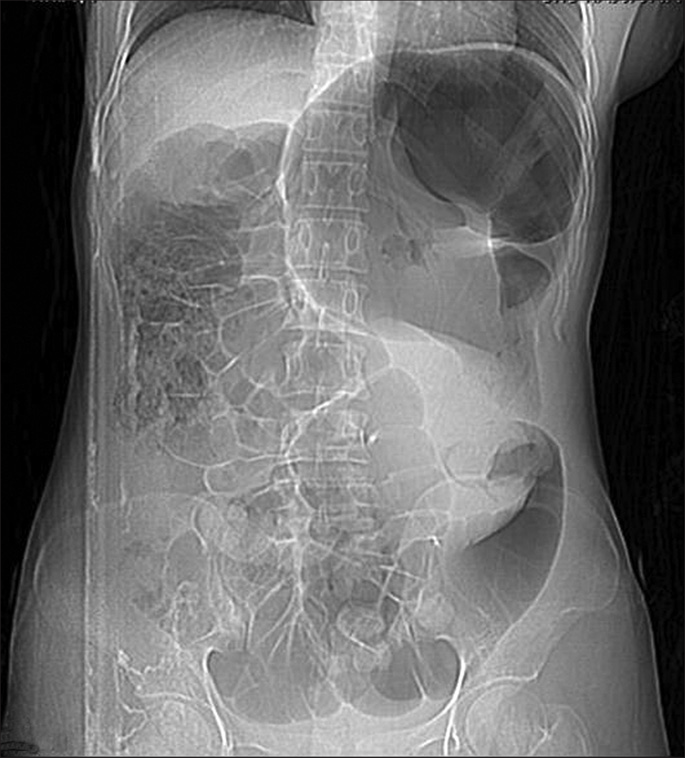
Abdominal radiography showed an important colon distention at the upper left quadrant without air fluid levels
